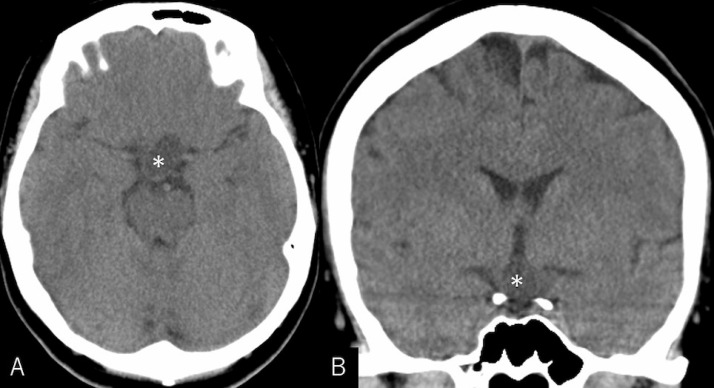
Noncontrast axial (A) and coronal (B) computed tomography scans showing a cystic mass in the suprasellar region (asterisk). It appears as a homogenous hypodense mass lacking intralesional calcifications.
